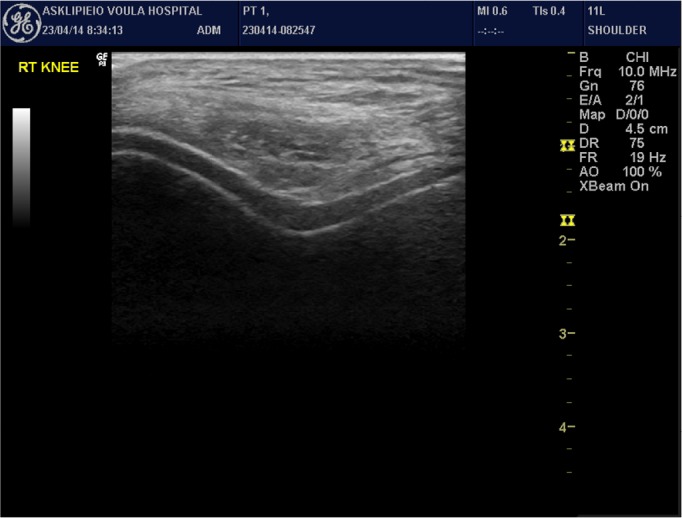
Patient 2. Ultrasound of knee cartilage with double contour sign.
